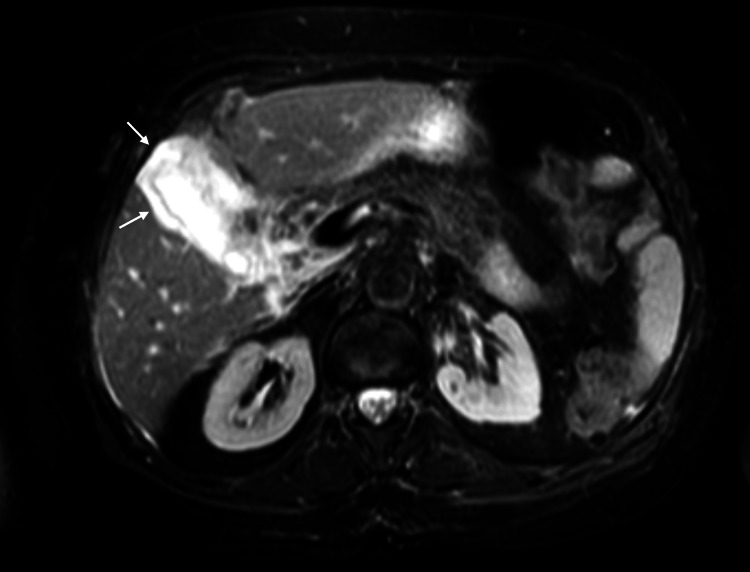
Axial T2-weighted fat-suppressed cholangioresonance showing diffuse gallbladder wall thickening (arrows) without intraluminal stones.
